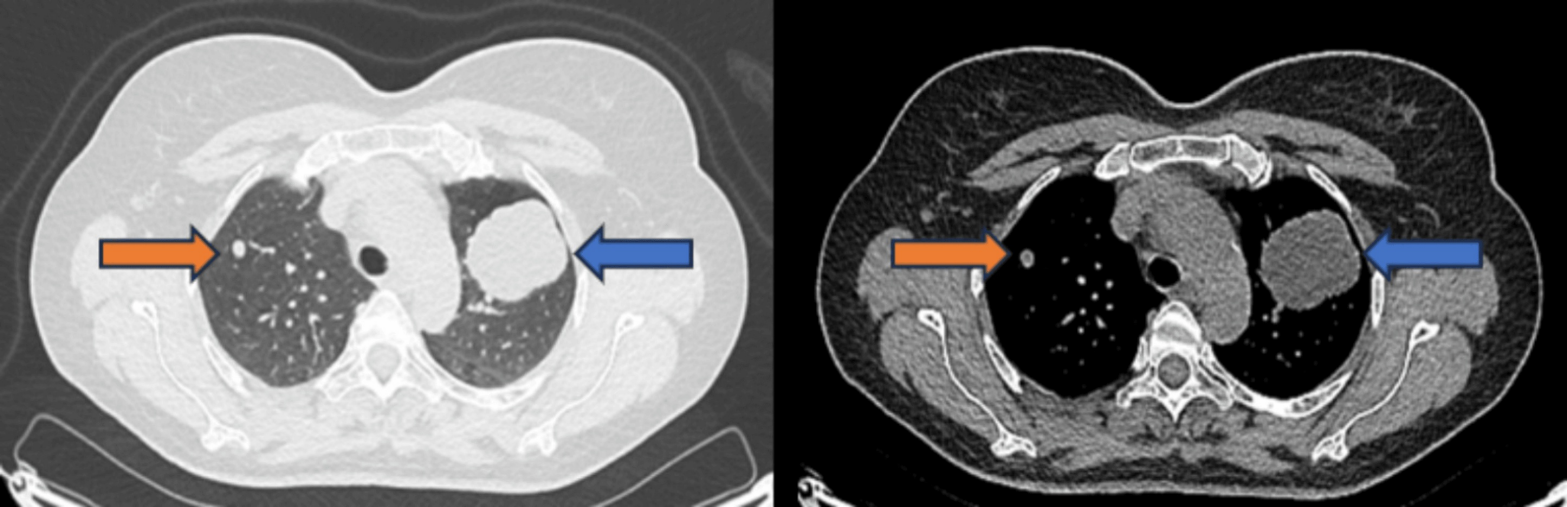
CT scan obtained at the time of the patient’s presentation showing a mass in the left upper lobe measuring 4.0 x 5.0 x 5.2 cm (blue arrow) and a nodule 0.3 cm in the right upper lobe (orange arrow)
